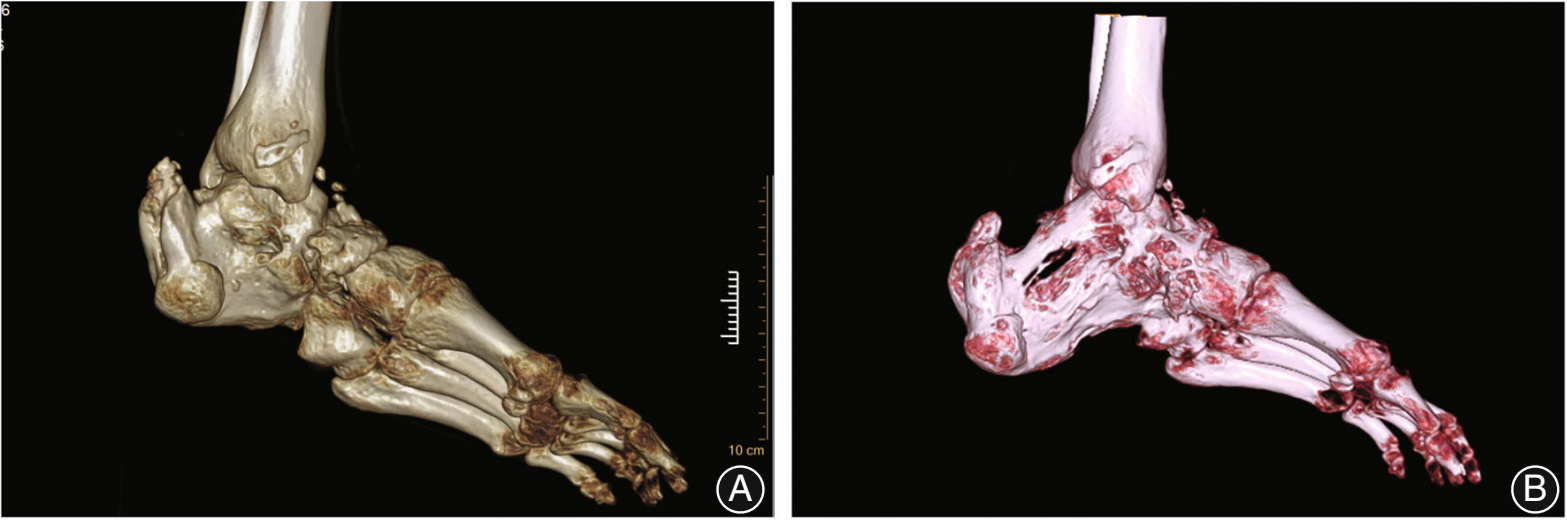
Three‐dimensional reconstruction of the affected foot based on computed tomography. (A) Preoperative appearance of the affected foot after three‐dimensional reconstruction. (B) Alignment of the involved ankle and foot was ultimately reconstructed.
